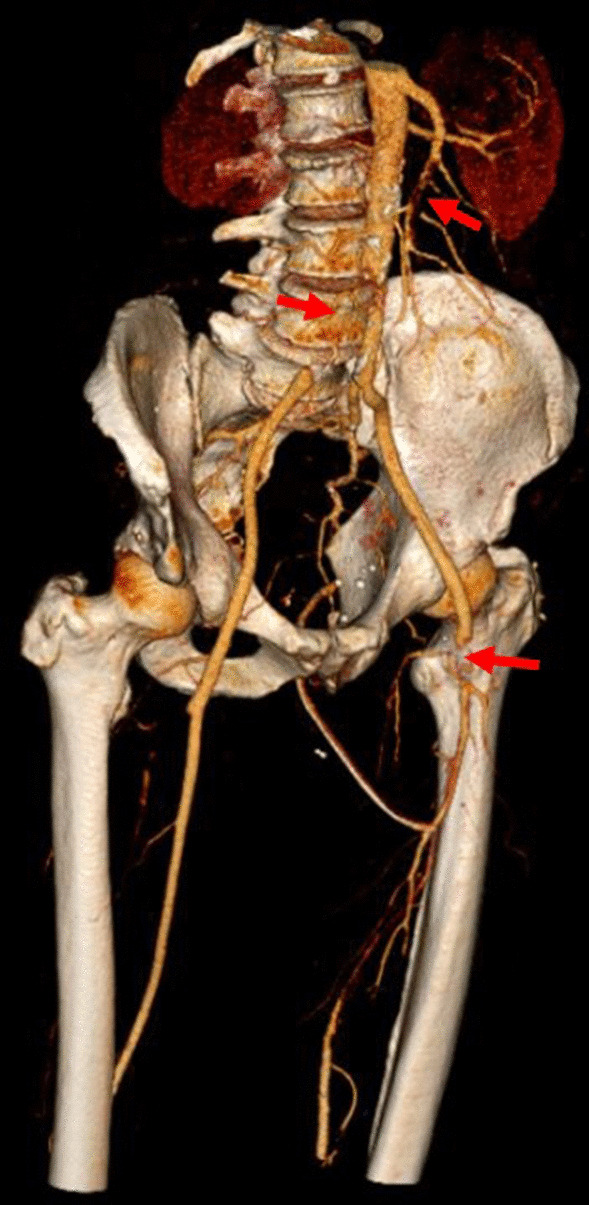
3D-Reconstruction of CT images showing several arterial thrombotic embolies with incomplete vascular occlusion. Red darts indicate a branch of the upper mesenterial artery, the right common iliac artery and the left femoral artery from top to bottom
